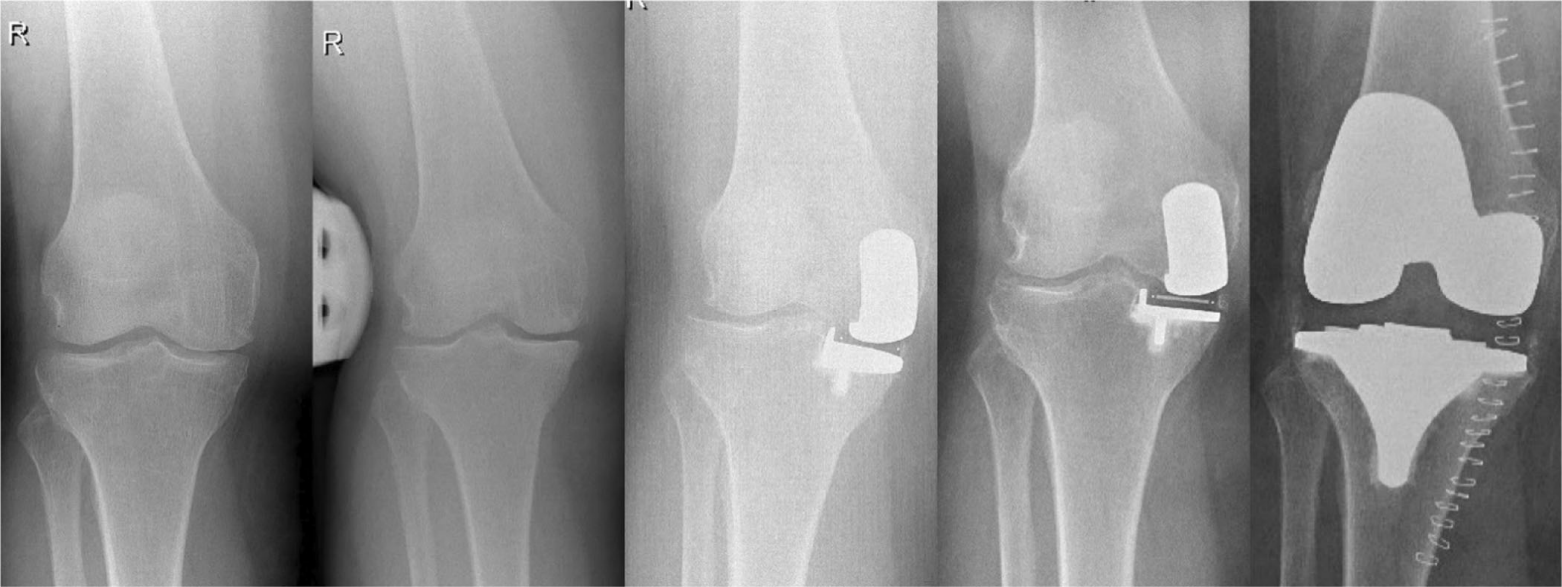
Preoperative radiographs show near bone‐on‐bone medial osteoarthritis (OA), and possibly slight joint space narrowing of the lateral compartment on the stress radiograph. The post‐operative radiograph shows no abnormalities. The pre‐revision radiograph shows lateral OA progression.
